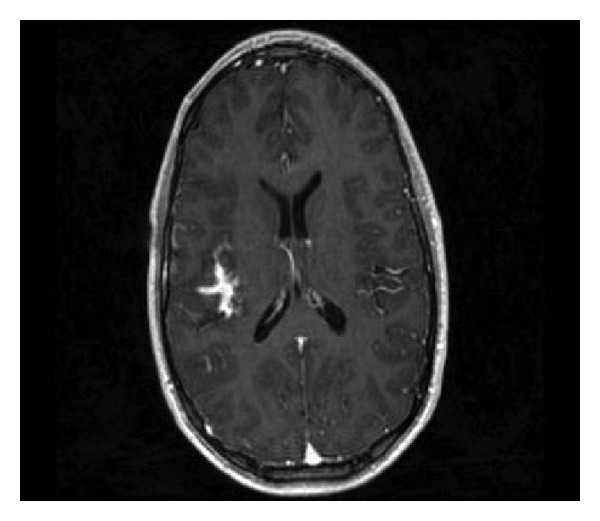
Relatively T1-weighted gradient echo axial MRI with gadolinium contrast shows serpentine enhancement in depths of right sylvian fissure compatible with presumed vascular malformation.
